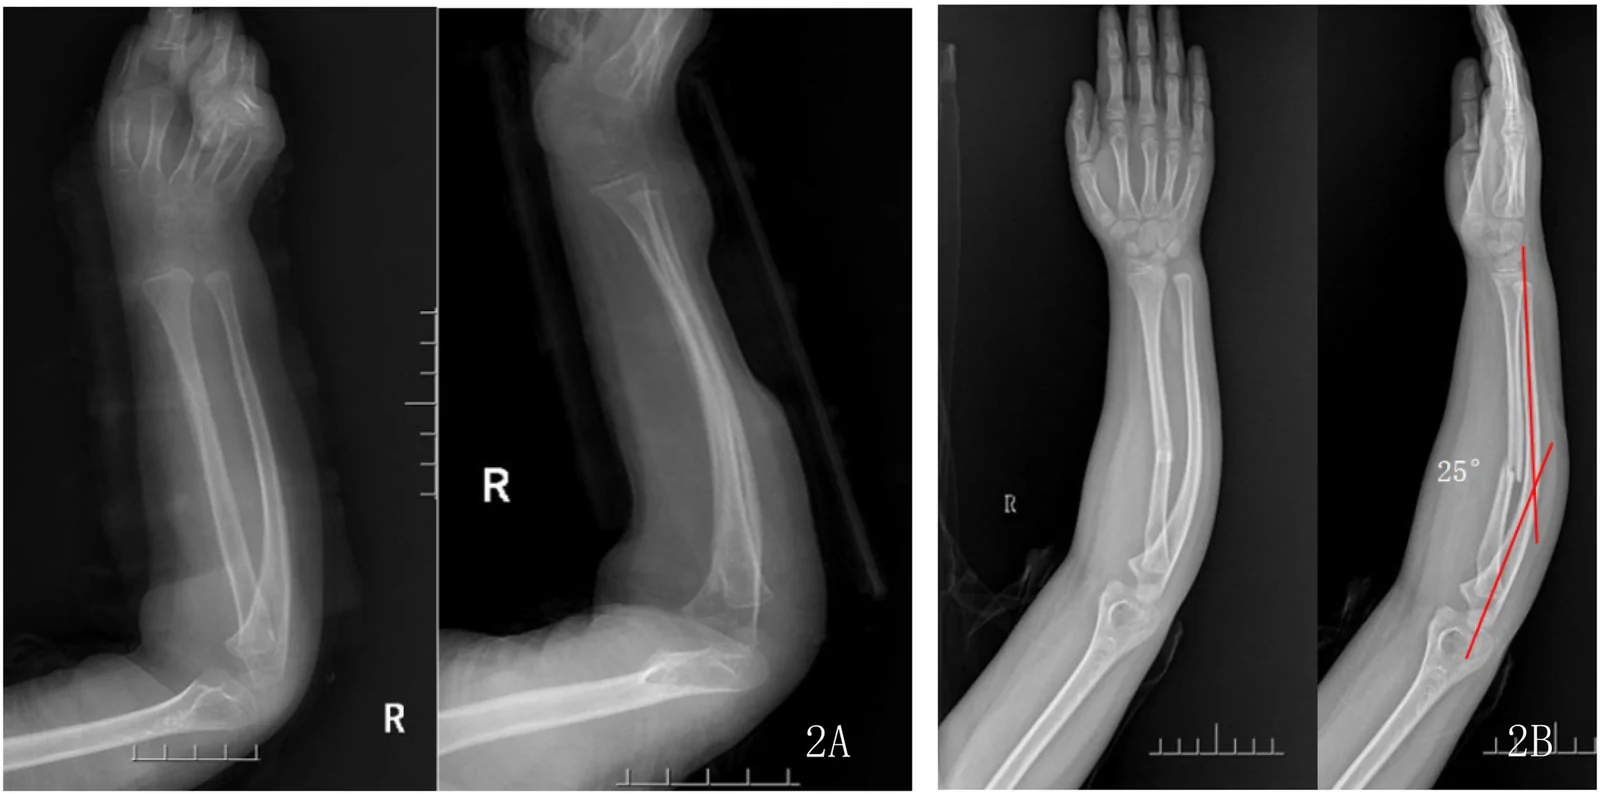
Four-week follow-up radiograph after small splint external fixation for the initial radial fracture (A); right forearm radiograph after recurrent fall trauma four years later, showing persistent ulnar bowing deformity (B).
